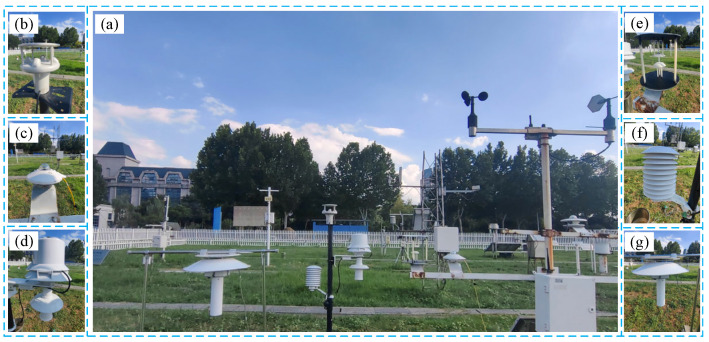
(a) Radiation-induced temperature deviation test platform; (b) ultrasonic anemometer; (c) CMP10 solar radiation sensor; (d) Model 43502 forced-ventilation temperature observation system; (e) proposed system; (f) Model 41003 naturally ventilated temperature observation system; (g) Model 076B forced-ventilation temperature observation system.
